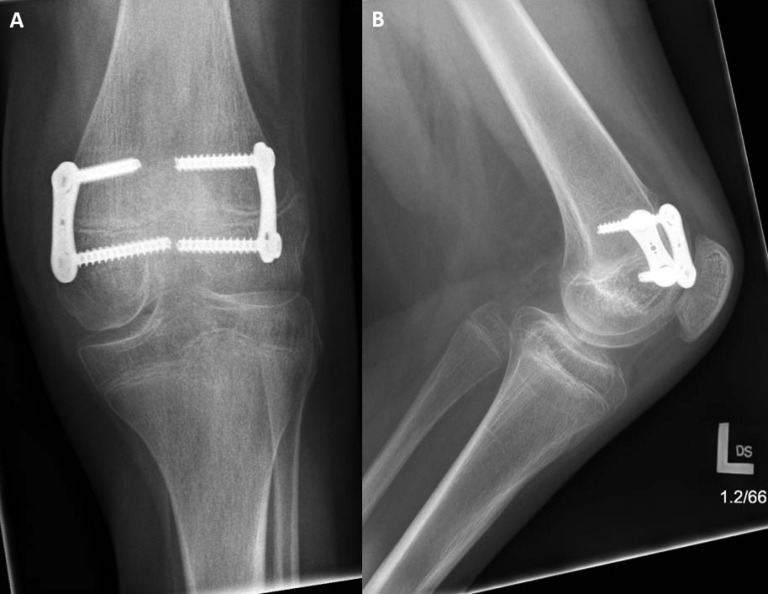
A, An AP radiograph of the patient's left knee demonstrating placement eight-plates postoperatively. B, A lateral radiograph of the patient's left knee, showing the eight plates from a different angle.
